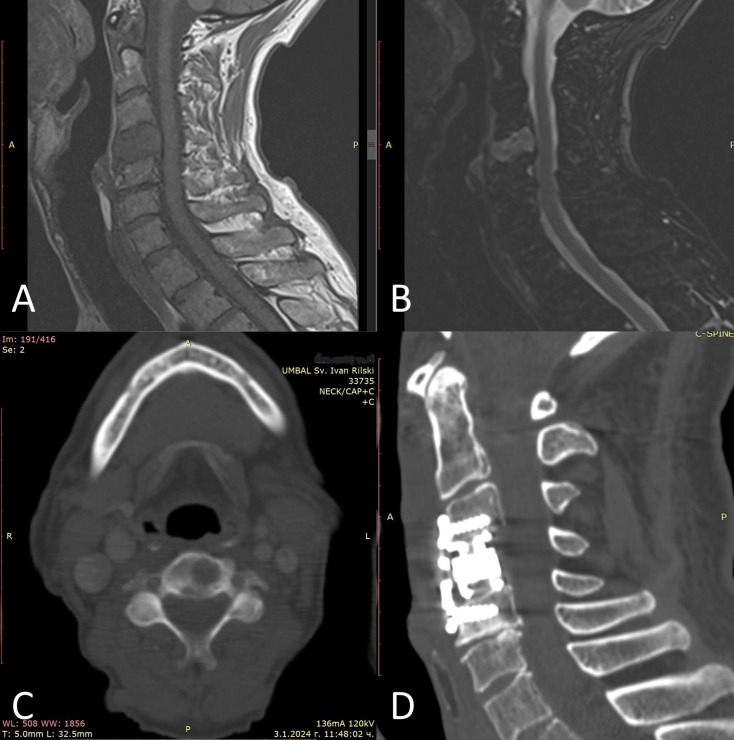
Magnetic resonance imaging of the cervical spine indicates a malignant disseminated process in the C4 vertebra, with characteristics of a metastatic lesion. (A) T1 sagittal sequence; (B) T2 TIRM (Turbo Inversion Recovery Magnitude) sagittal sequence; (C) Preoperative axial CT scan image demonstrating osteolytic vertebral lesions; (D) Postoperative sagittal CT scan image showing corpectomy and vertebral column stabilization.
